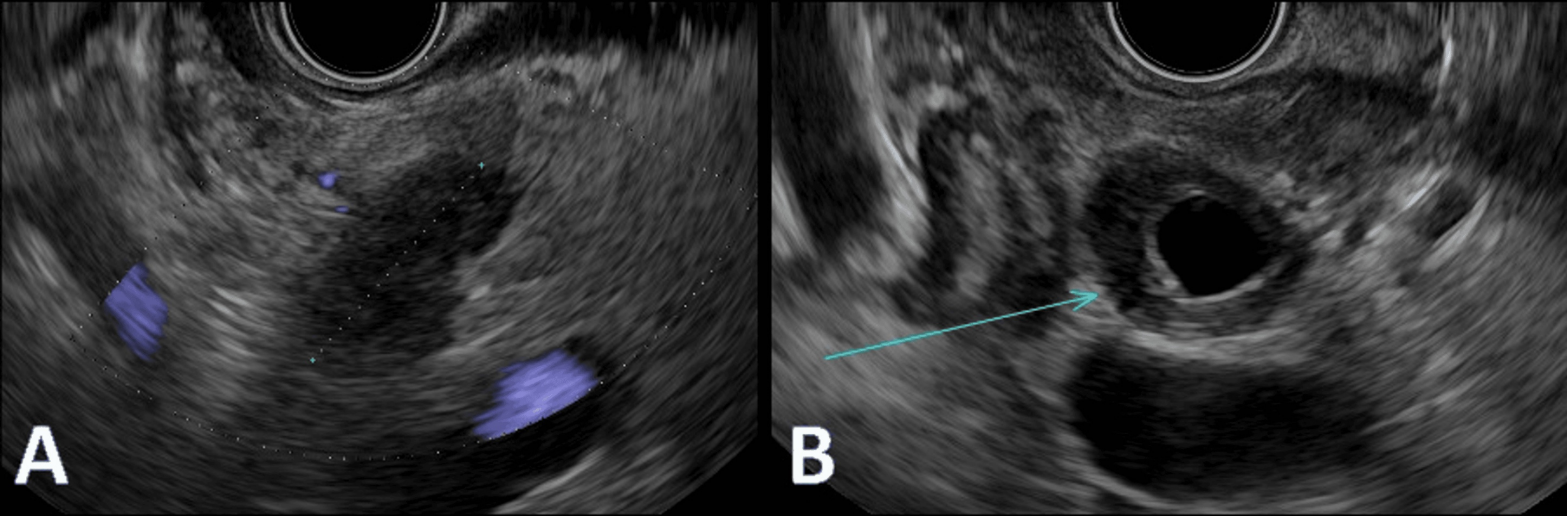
Endoscopic ultrasound. (A) A heterogeneous lesion at the pancreatic head. (B) Diffuse common bile duct thickening (blue arrow).
